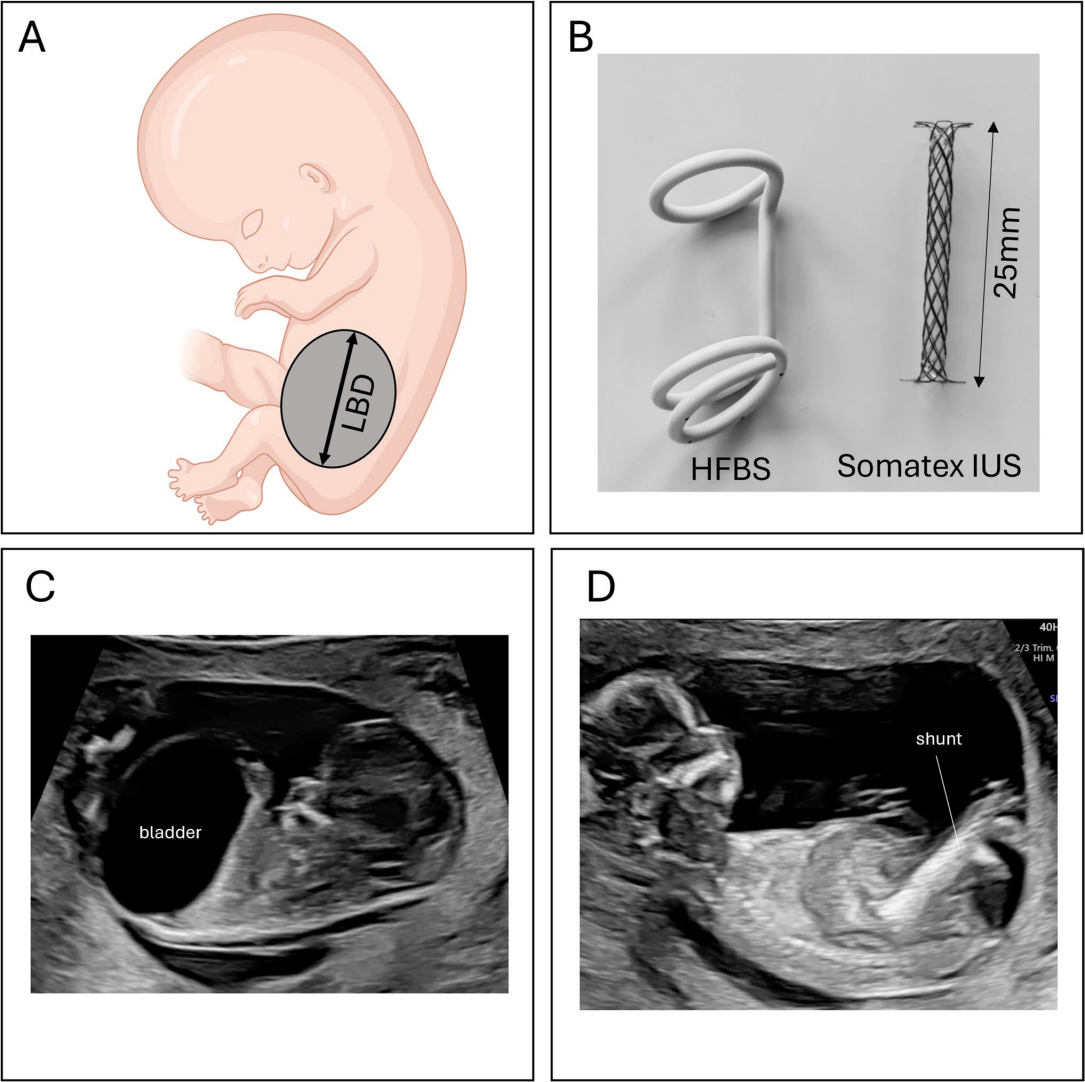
Fetal ultrasonography in congenital lower urinary tract obstruction. A Measurement of longitudinal bladder diameter in a first-trimester fetus with megacystis. B Image of two commonly used vesico-amniotic shunt systems: Harrison Fetal Bladder Stent (HFBS; left) and Somatex® intrauterine shunt (IUS; right). The shunts are deployed in the picture. C Ultrasound picture of a fetus with first-trimester megacystis (image courtesy of Eva C. Weber). D Ultrasound picture of a fetus after first-trimester vesico-amniotic shunting with the Somatex® IUS showing a completely drained bladder (image courtesy of Eva C. Weber)
